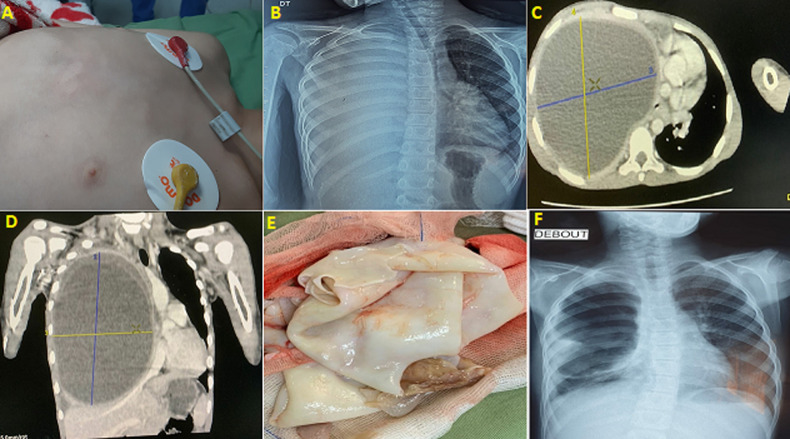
A) preoperative image showing the bulging aspect of the right hemithorax; B) standard radiograph showing the appearance of right pleurisy; C) computed tomography scan in axial sections showing the right huge; D) computed tomography scan in frontal sections showing the right huge; E) intra-operative image of the proligeral membranes; F) standard control radiograph showing the re-expansion of the right lung
